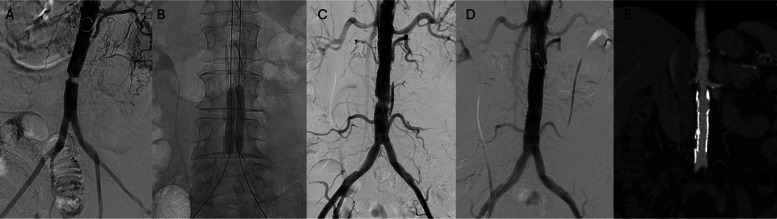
A Baseline DSA demonstrating extensive atherosclerotic disease with significant aortic stenosis, B Kissing intravascular lithotripsy (IVL) balloon technique applied across the lesion., C Post-IVL DSA revealing aortic dissection., D Covered stent implantation with restoration of luminal integrity and no residual stenosis., E Six-month follow-up CTA demonstrating no residual stenosis
